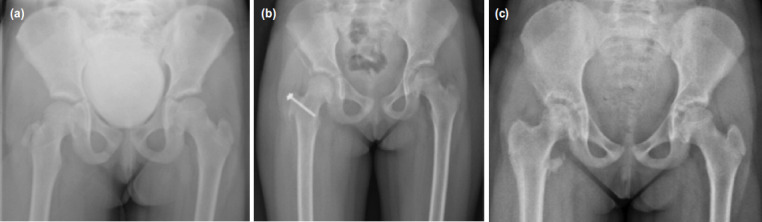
(a) Anteroposterior radiographs of the right hip at the time of presentation, (b) after surgery (c) and after screw removal.
